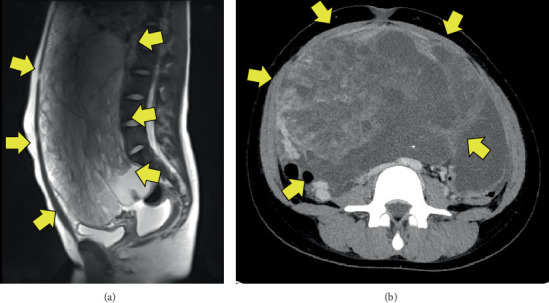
(a) Sagittal section of magnetic resonance imaging showing a giant abdominopelvic mass (primary ovarian tumor). (b) Axial computed tomography image demonstrating the same mass.
